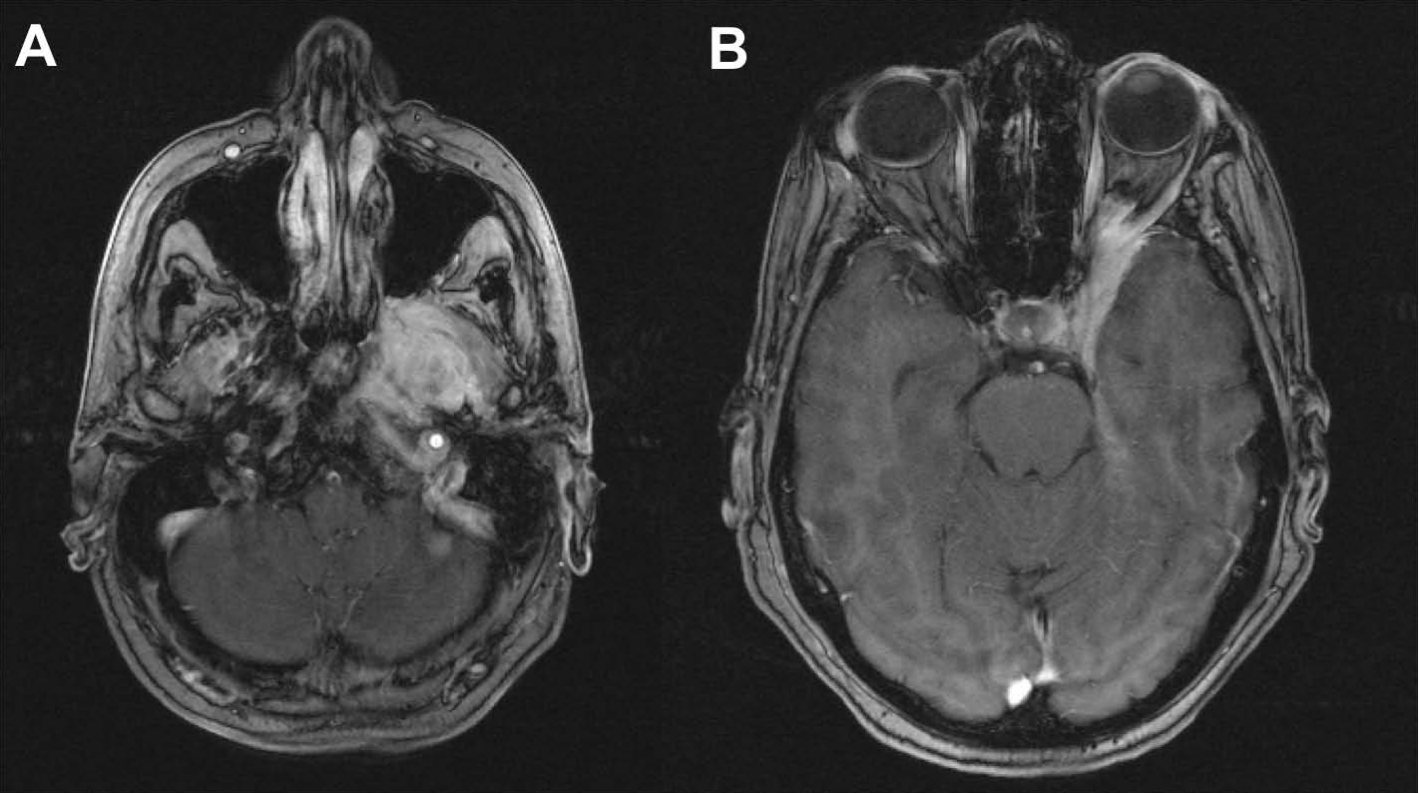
Initial, contrast-enhanced MRI of a large adenoid cystic carcinoma extending from the left pterygoid muscles (a) into the cavernous sinus and left orbit (b).
